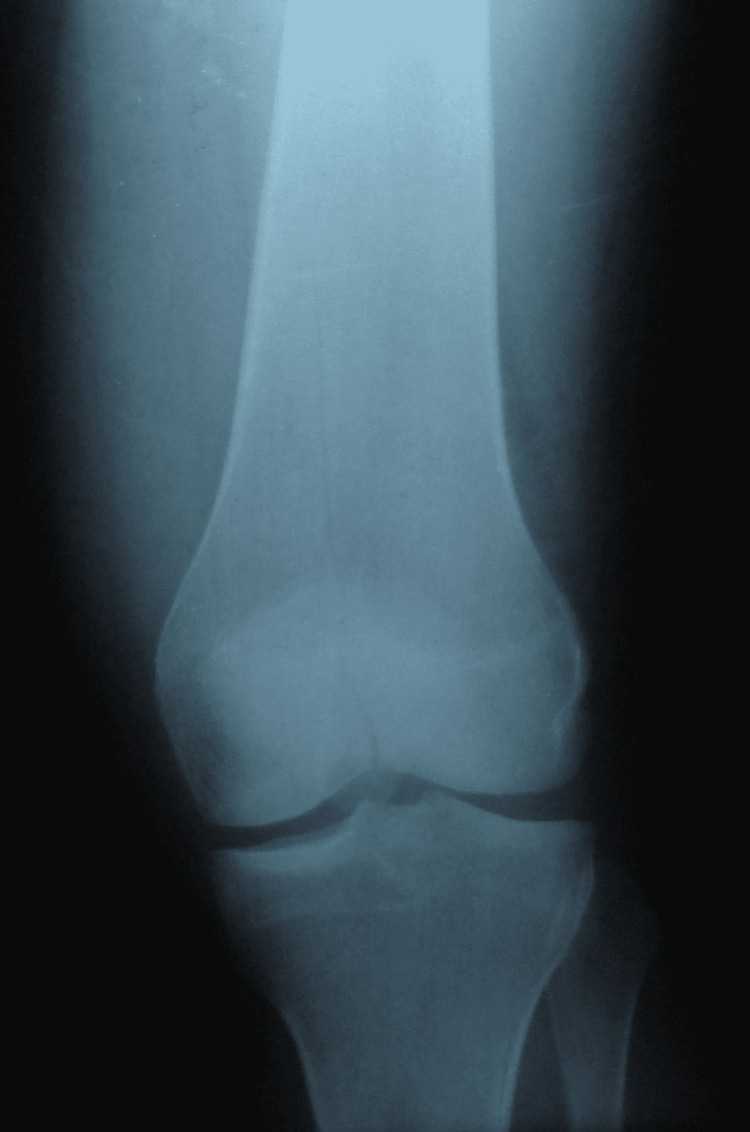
Plain radiograph showing a vertical fracture line of the femoral metaphysis extending to the intercondylar notch without separation of a bone fragment
